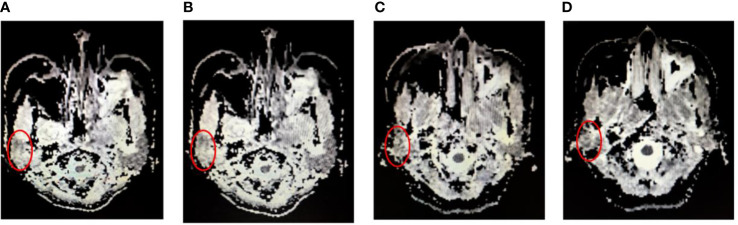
Typical DWI image for parotid glands (red circles) during radiotherapy. (A) at pre-radiation, (B) at 15^th^ radiation, (C) at 25^th^ radiation and (D) at completion of radiation.
